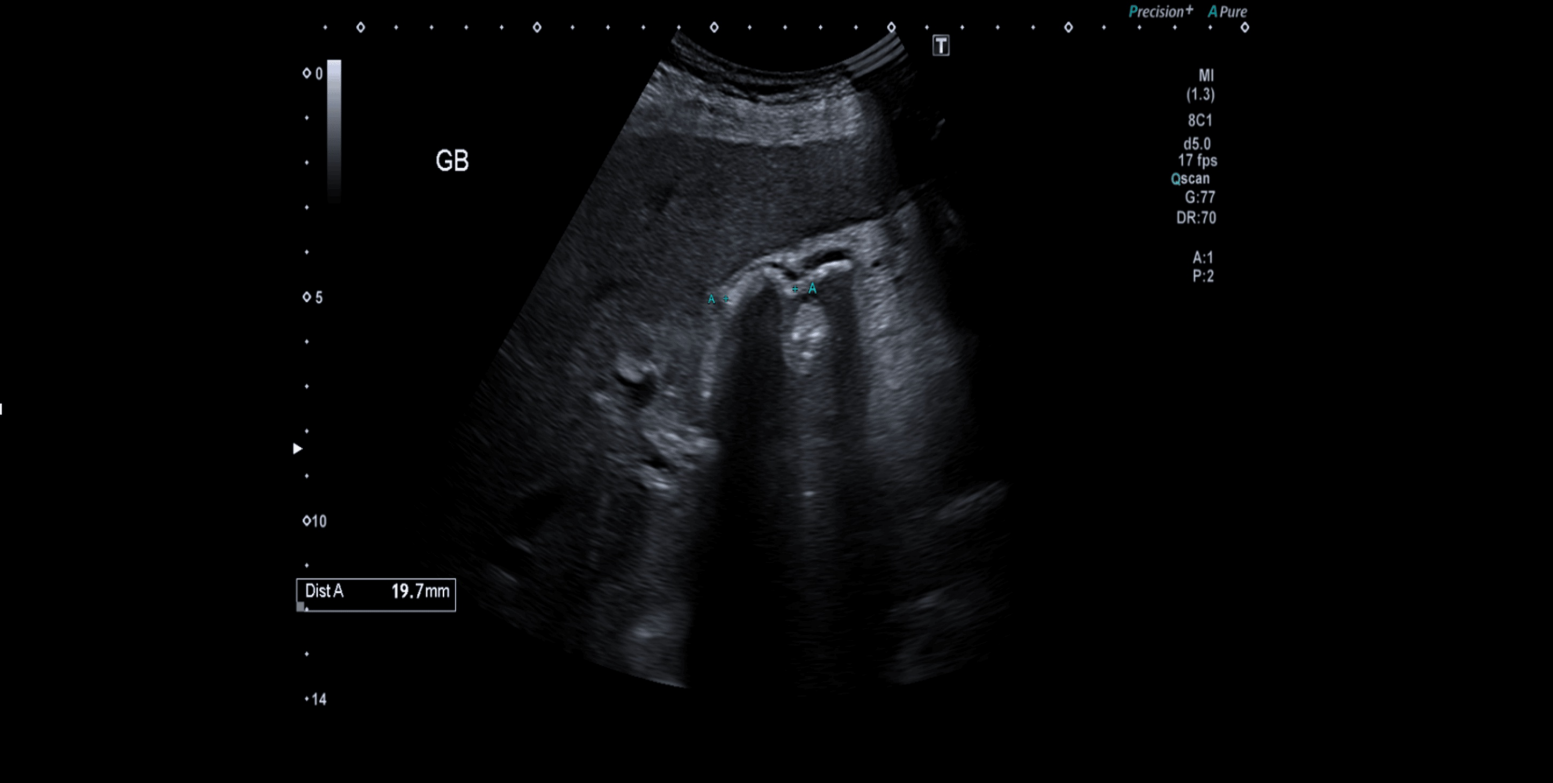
Abdominal ultrasound image of the patient on initial presentation demonstrating a thin-walled gallbladder without evidence of cholecystitis.
